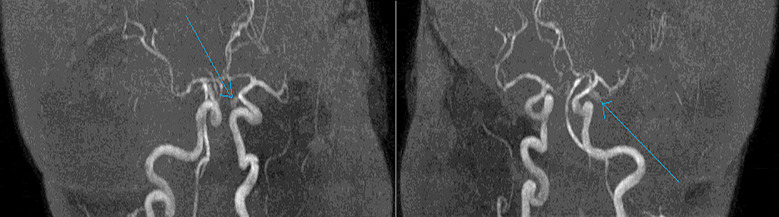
rotated sagittal views of arterial phase on CT angiogram demonstrating the left posterior communicating artery aneurysm (blue line arrow)
